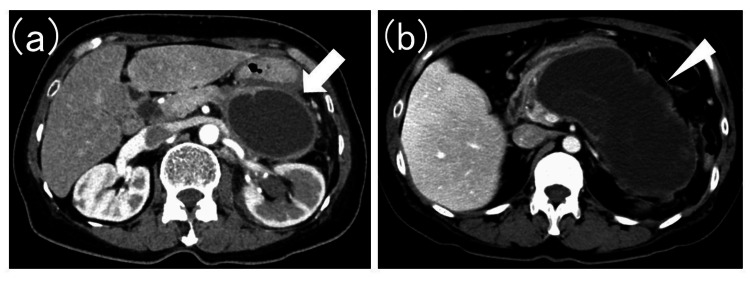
Computed tomography images of pancreatic pseudocyst and walled-off necrosis (a) Pancreatic pseudocyst (white arrow). (b) Walled-off necrosis (white arrowhead).
